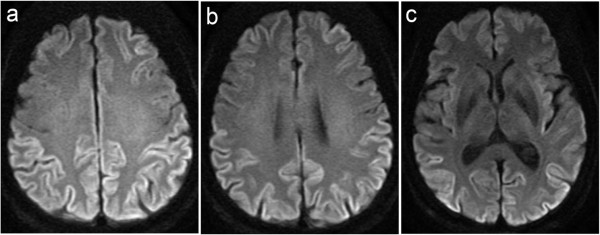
Axial sections of the diffusion-weighted magnetic resonance images of the brain. These images (a-c) reveal cortical hyperintensity in the bilateral parietal, occipital, and temporal lobes.
